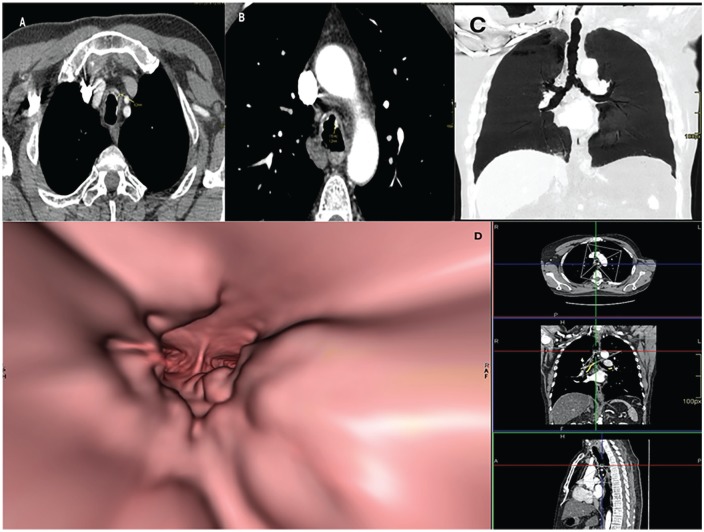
(A) Thoracic axial computed tomography (CT) scan with moderate nodular thickening of the anterior and lateral walls of the trachea of heterogeneous density and scalloped edges. Note the absence of compromise of the posterior wall of the trachea. (B) Thoracic axial CT scan with multiple calcified endotracheal nodules (170 HU). (C) Thoracic coronal CT scan maximal intensity projection reconstruction showing endobronchial nodules and beaded appearance of the trachea. (D) Virtual bronchoscopy showing multiple nodules giving a cobblestone appearance with compromise extending to the level of the tracheal carina, as shown by the axial, coronal, and sagittal reconstructions at the right.
